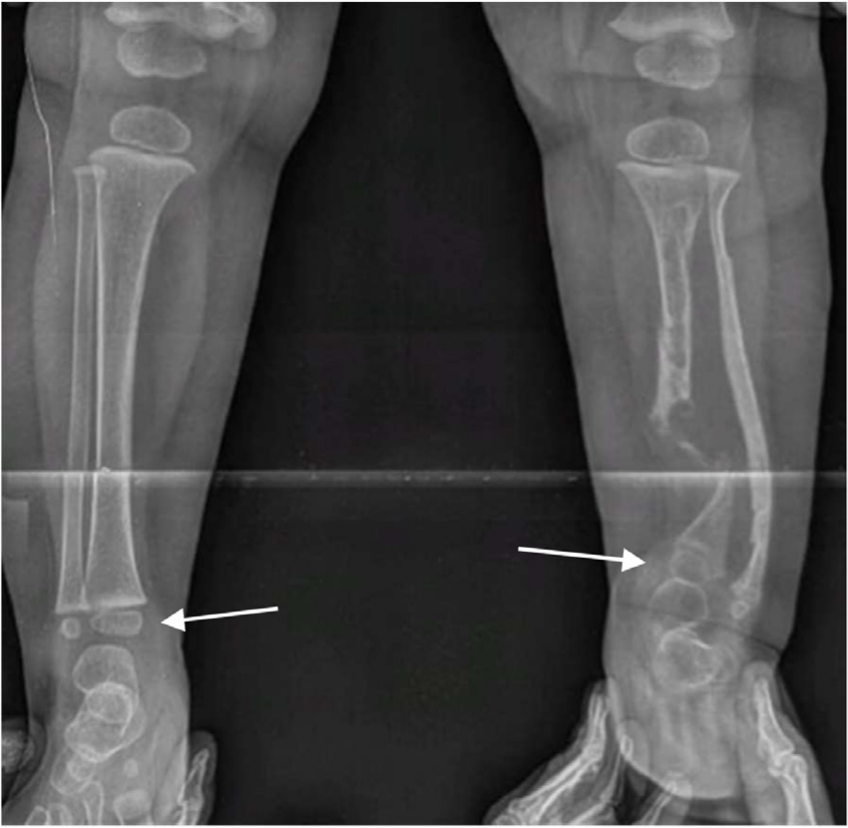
Lower limbs X-rays, performed during follow-up, show left leg 1.5 cm shorter than right (arrows).
